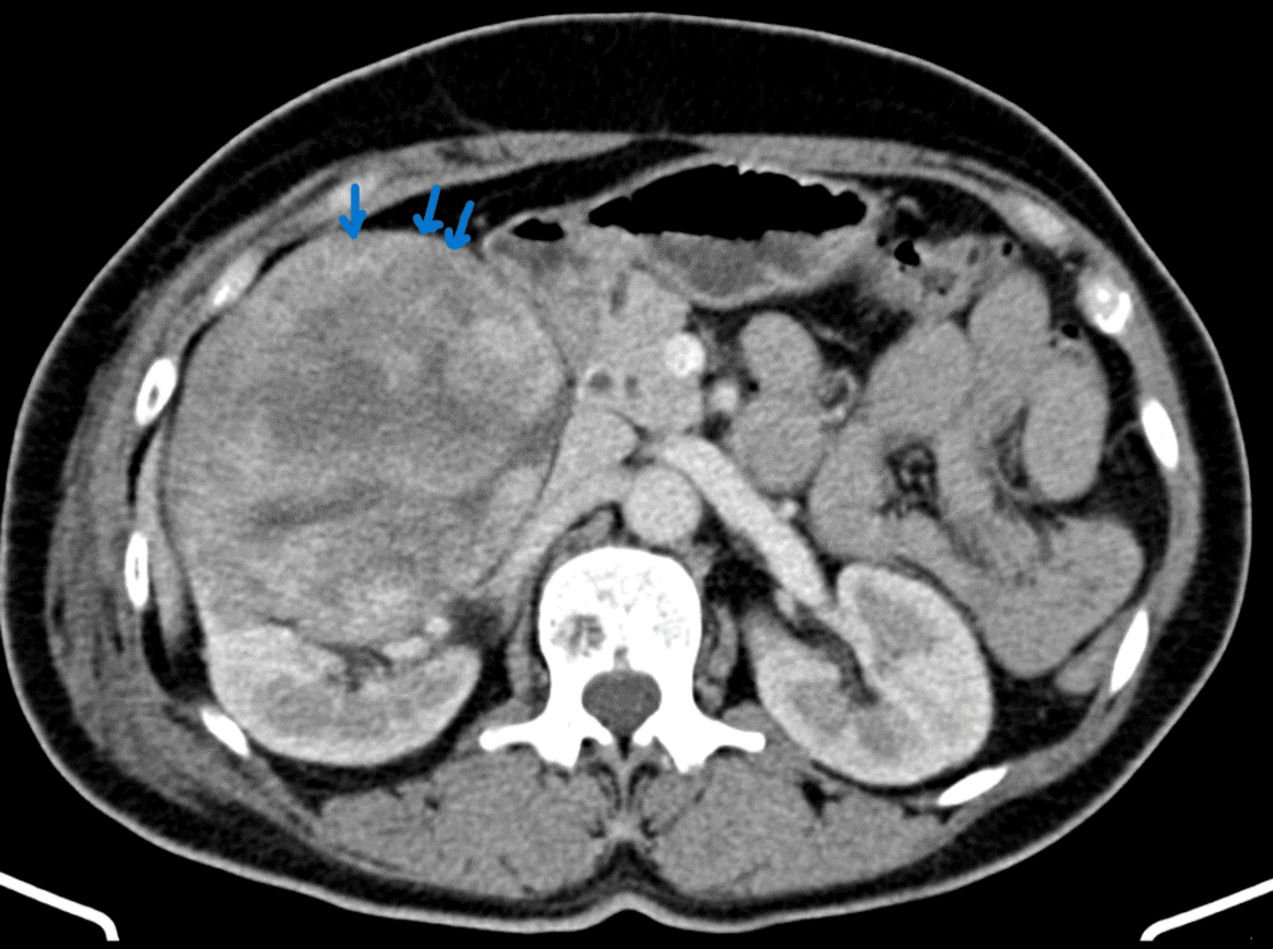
CT slice showing a well circumscribed mass of the right kidney of heterogeneous density (blue arrows).
